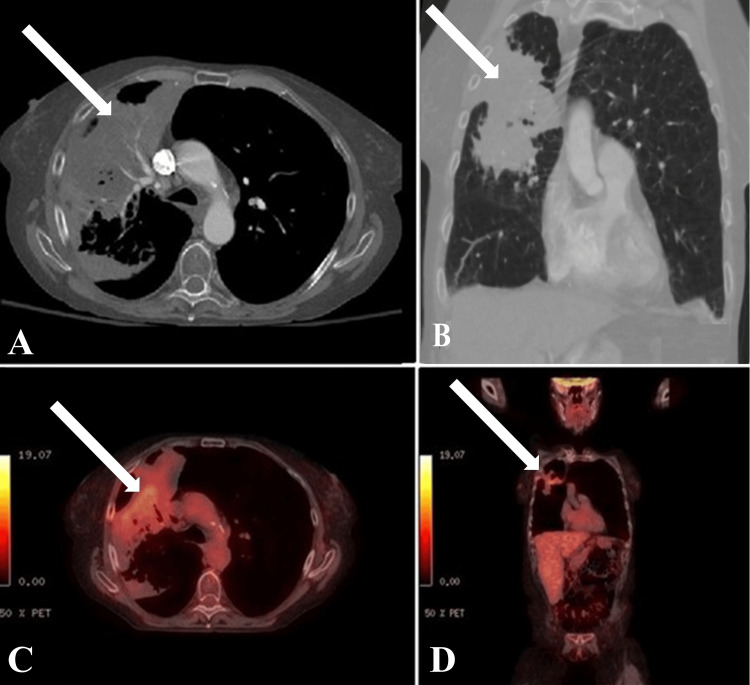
Follow-up imaging post treatment (A) Axial and (B) coronal views of the follow-up chest computed tomography scan. (C) Axial and (D) coronal views of the follow-up positron emission tomography-computed tomography scan.
